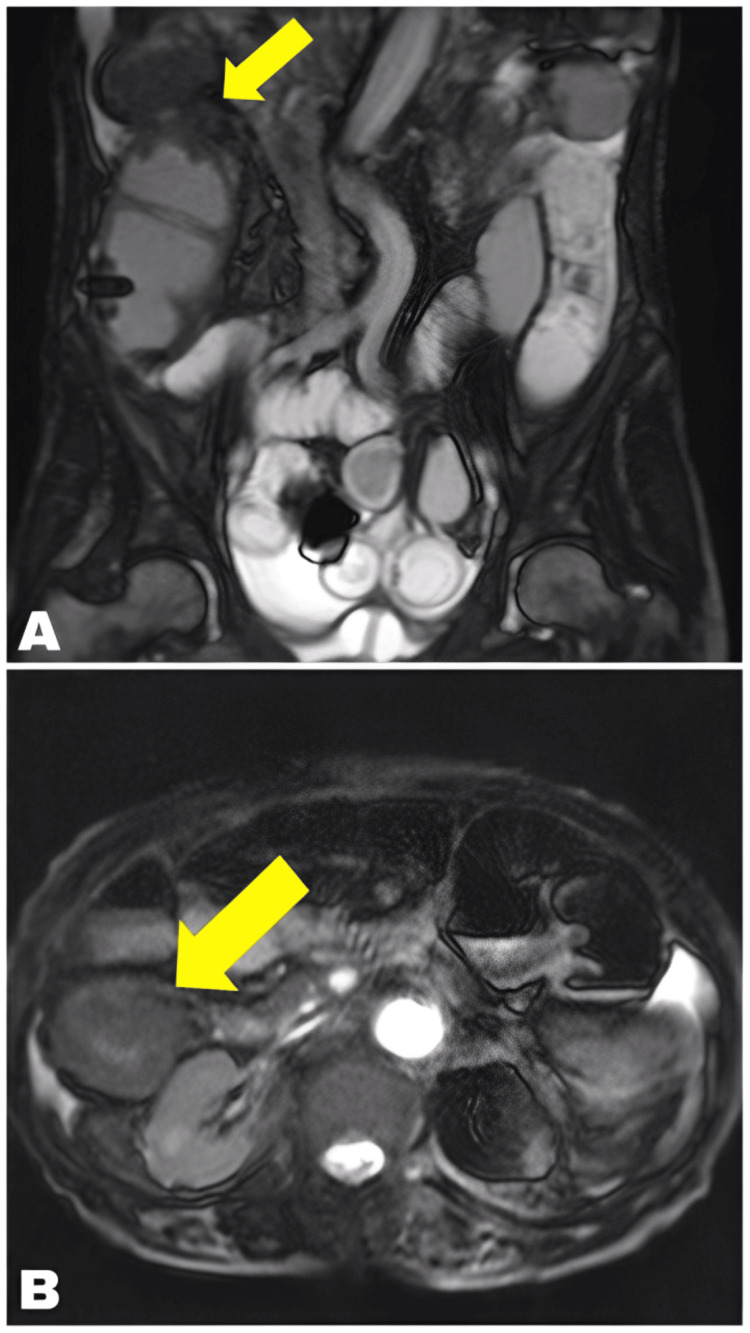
MRI of ascending colon cancer at admission MRI shows tumor occlusion and irregular wall thickening in the ascending colon near the hepatic flexure, along with proximal intestinal tract distension.
